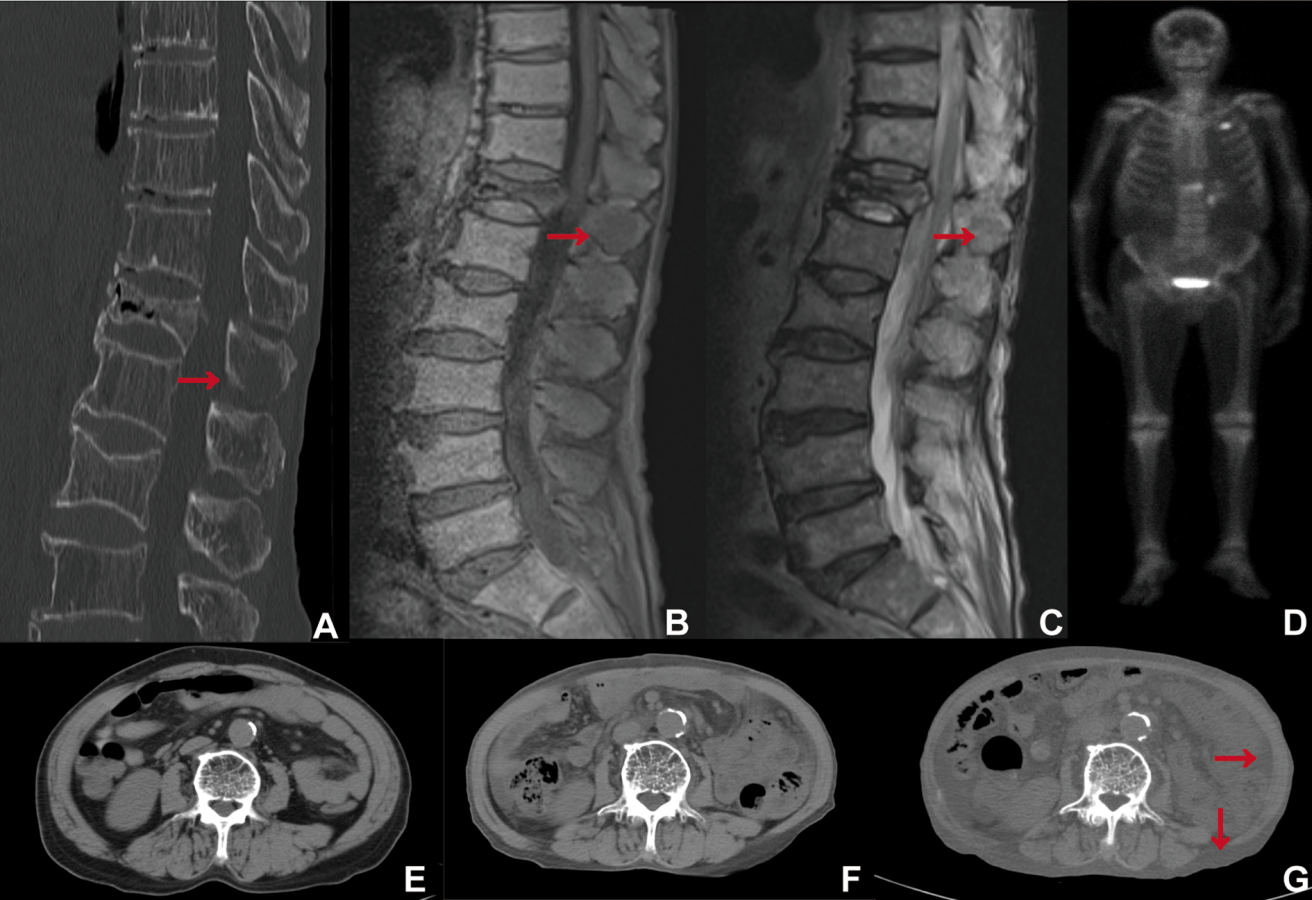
(A) Thoracolumbar spine CT (WW/WL 2600/800), (B) T1WI, (C) fat suppressed T2WI (T2WI-STIR), (D) Radionuclide bone scintigraphy, (E–G) Abdominal CT scans were performed 24 months prior (E), 18 months prior (F), and one month prior (G), with a WW of 350 and a WL of 40.
